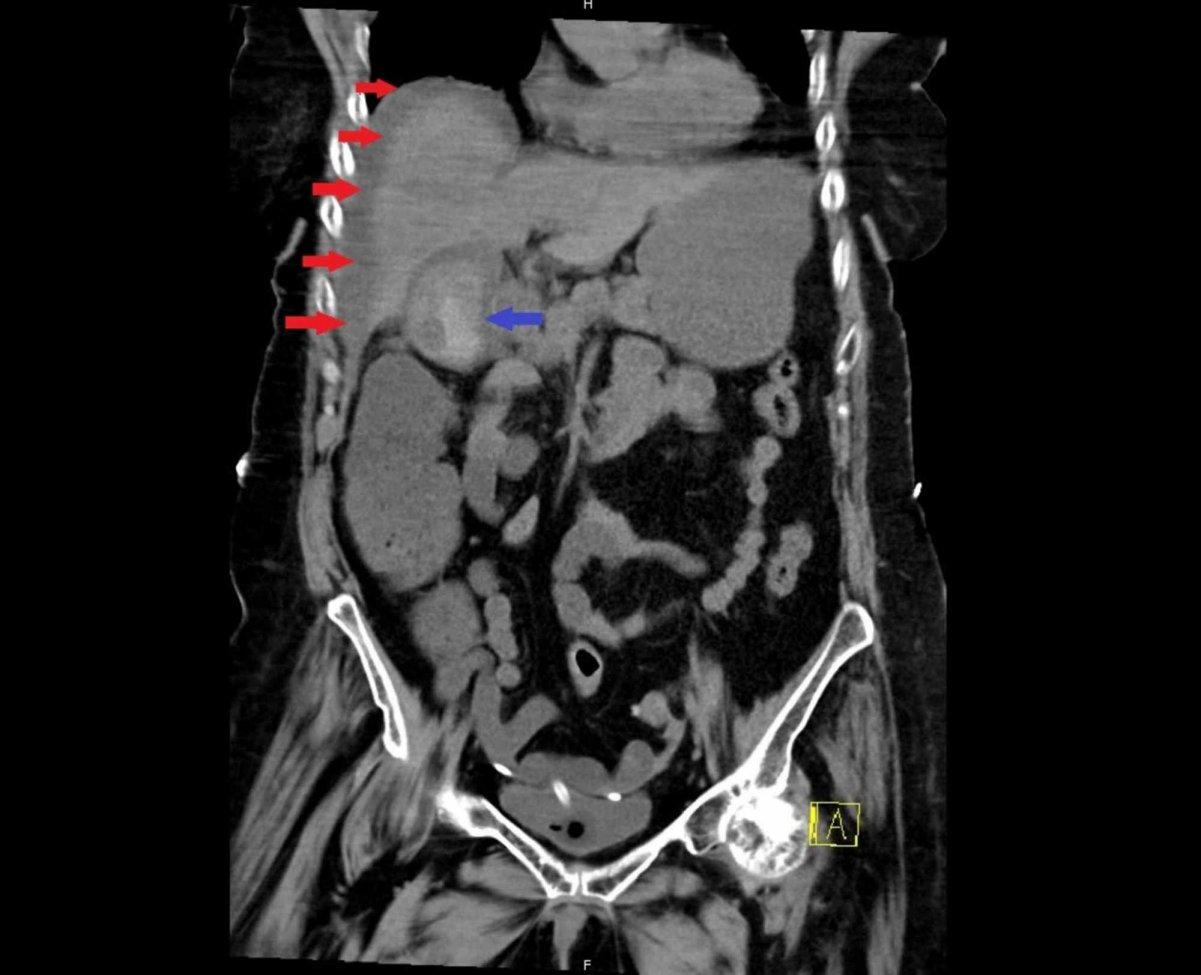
CT scan of the abdomen and pelvis showing perihepatic fluid (red arrows) secondary to acute cholecystitis complicated by gallbladder perforation (blue arrow).
